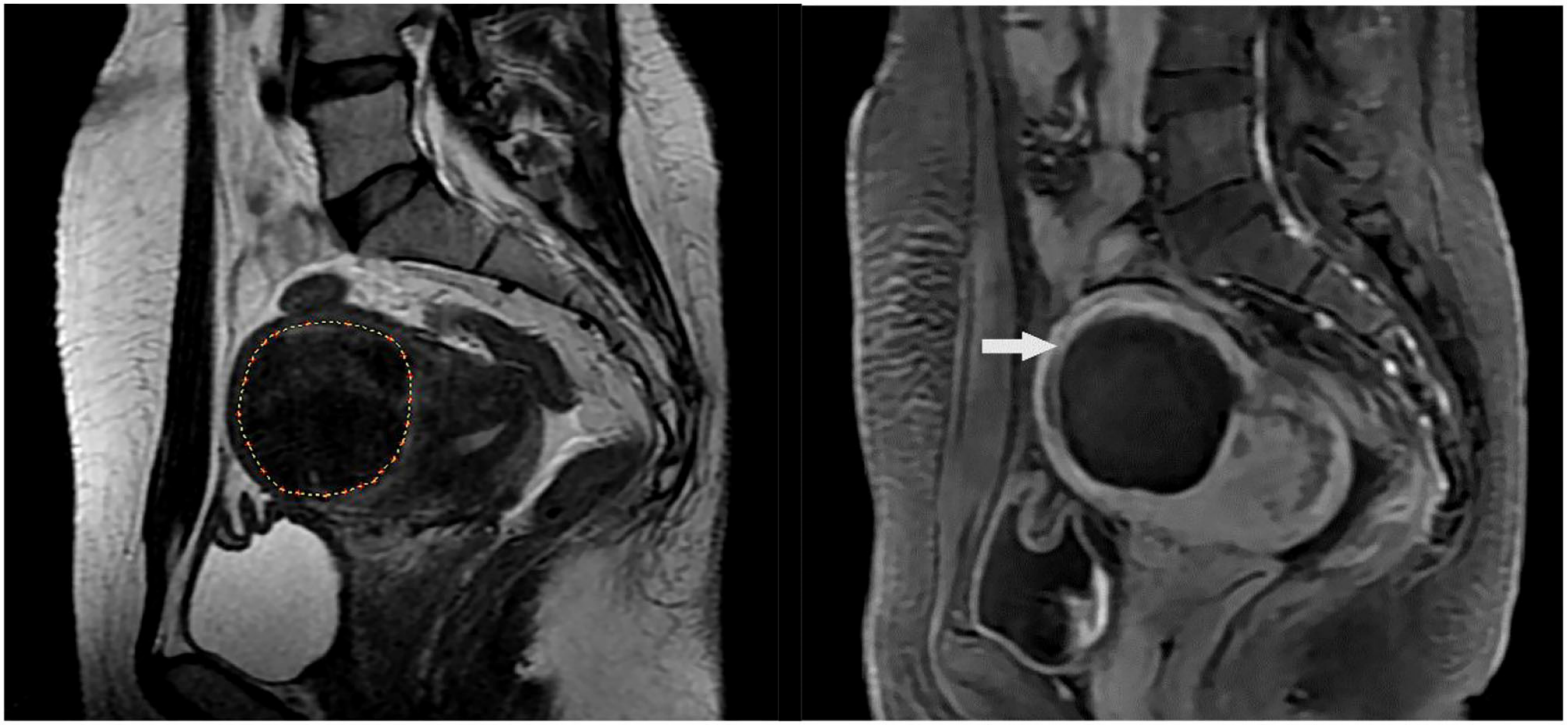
MR images of a 40-year-old woman with uterine fibroid. (A) T2-weighted image shows a single fibroid in the anterior wall of uterine before high-intensity focused ultrasound (HIFU) treatment; (B) Contrast-enhanced MRI shows nonperfused volume (NPV) in the uterine fibroid after HIFU treatment, NPV ratio (NPVR) was 94%. The white arrow indicates the nonperfusion volume (NPV) after treatment.
